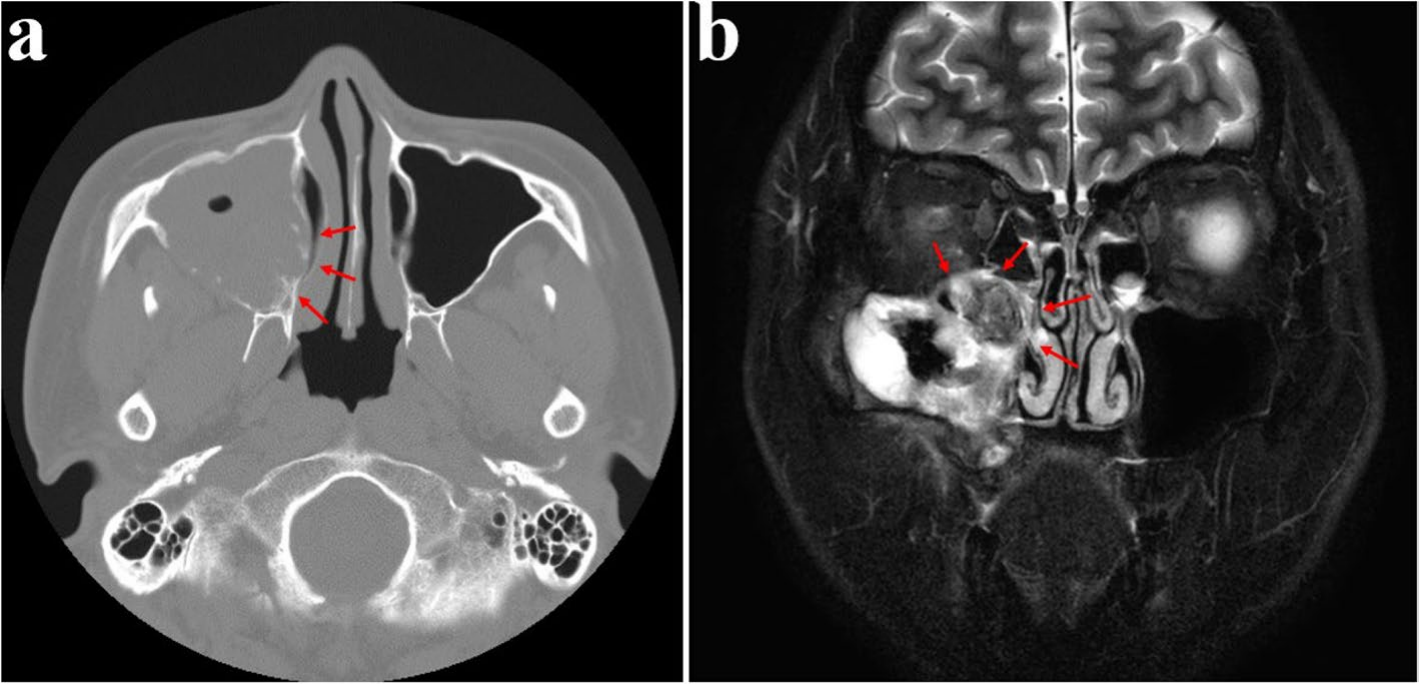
Four-month postoperative CT reveals residual tumor near the right ostium, (a) CT PNS (enhanced) axial view, (b) MR PNS (enhanced) coronal view
